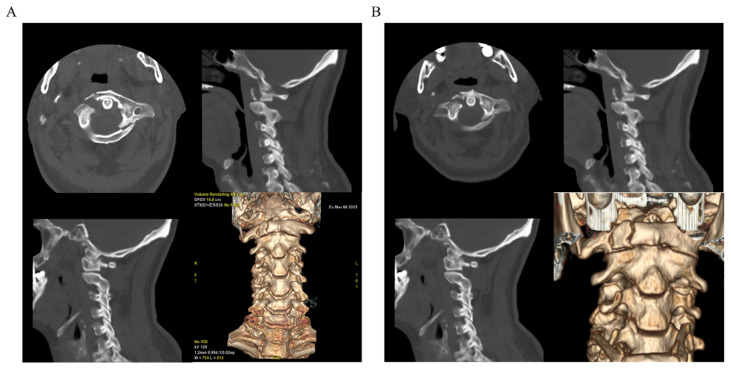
CT imaging of pre-traction (A) and after 8 kg traction (B).
